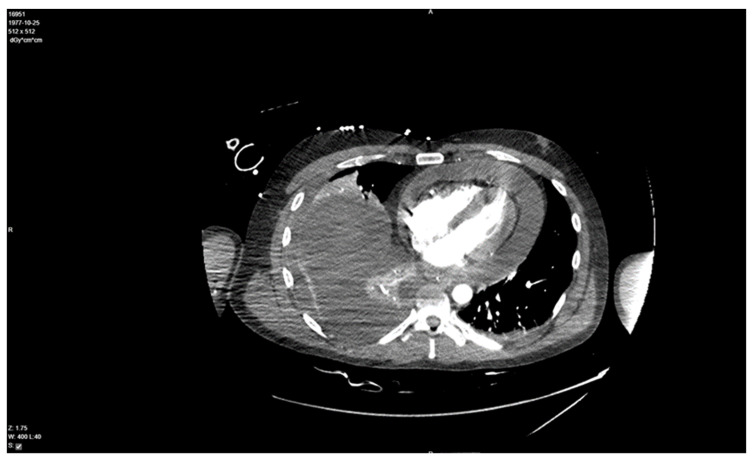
Chest CT demonstrates right-side effusion and a 26 mm thickness buildup of fluid in the pericardial space.
